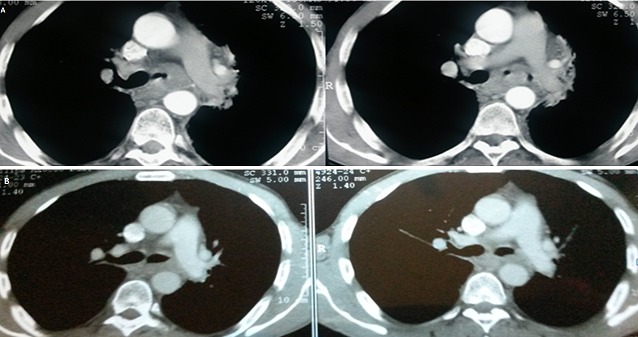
Transaxial CT scan showing a large polypoid intra-luminal mass involving the distal trachea and the carina, causing near total obliteration of the lumen. (A): Before radiotherapy, (B): After radiotherapy
